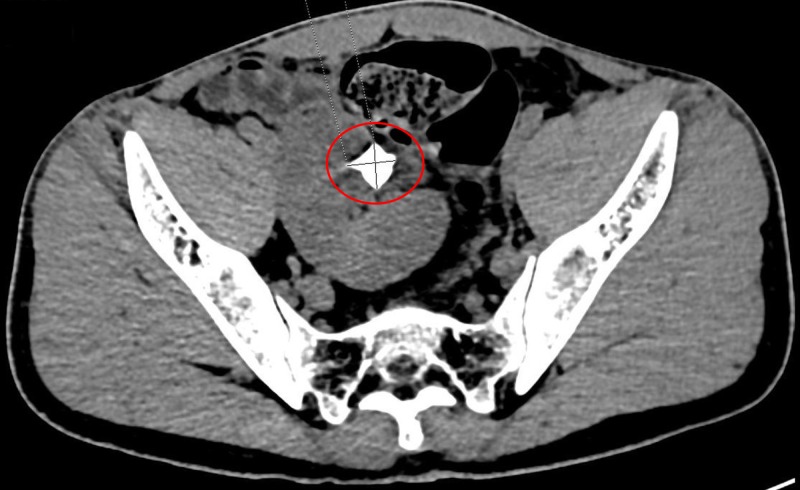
Non-contrast computed tomography of the abdomen revealing a left kidney on the right side fused to the lower pole of the right kidney.
